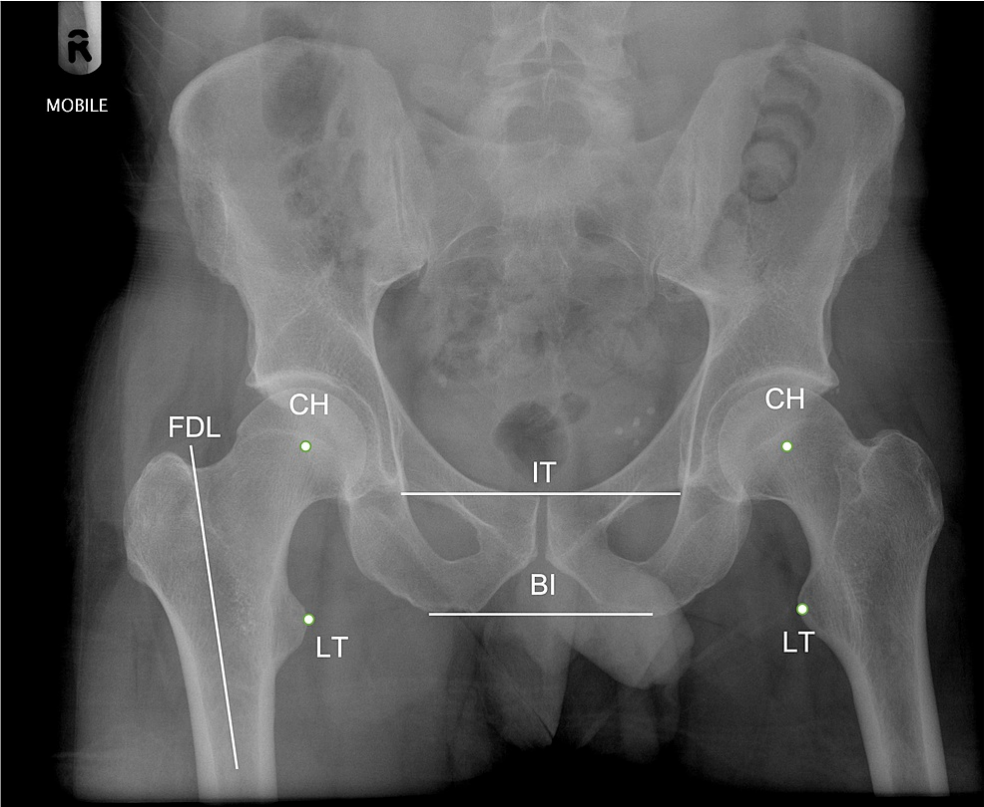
An anteroposterior (AP) radiograph of the pelvis shows the different landmarks marked to measure leg length discrepancy (LLD). CH = center of the femoral head; LT = tip of the lesser trochanter; BI = biischial line; IT = interteardrop line; FDL = Femoral Diaphyseal Line. Original radiograph image courtesy of Dr. Ian Bickle, Radiopaedia.org, rID: 37956
